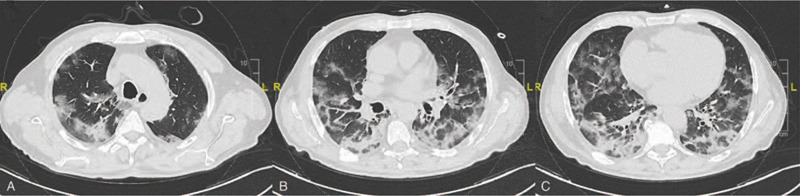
Exemplificative computed tomography scoring system on images of a 68 years old man with severe/critical disease. The total severity score is 53. It was calculated as: for upper level (A), right side (severity score: 9): 3 [mixed consolidation and GGO/crazy paving] × 3 [50–74% distribution] + left side (severity score: 2): 2 [GGO/crazy paving] × 1 [1–24% distribution]; for middle level (B), right side (severity score: 9): 3 [mixed consolidation and GGO/crazy paving] × 3 [50–74% distribution] + left side (severity score: 9): 3 [mixed consolidation and GGO/crazy paving] × 3 [50–74% distribution]; for lower level (C), right side (severity score: 12): 3 [mixed consolidation and GGO/crazy paving] × 4 [≥75% distribution] + left side (severity score: 12): 3 [mixed consolidation and GGO/crazy paving] × 4 [≥75% distribution]. GGO = ground glass opacity.
